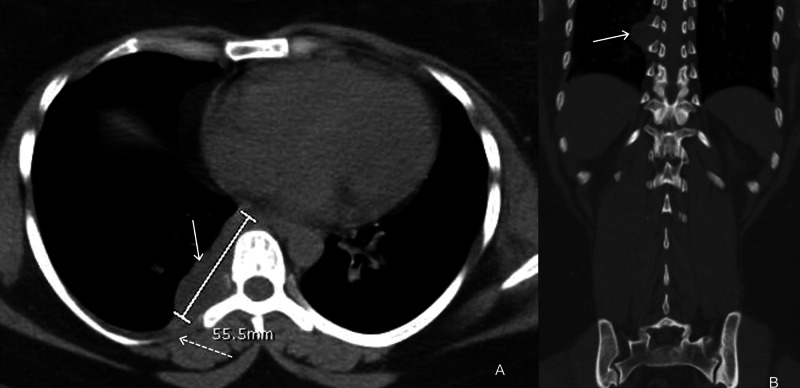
CT scan of the chest, axial (A) and coronal (B) images without contrast show a large 5.5 cm insinuating paravertebral mass (arrow) at the T7 and T8 vertebral bodies with smooth remodeling of the undersurface of the right seventh costovertebral junction (dashed arrow).
